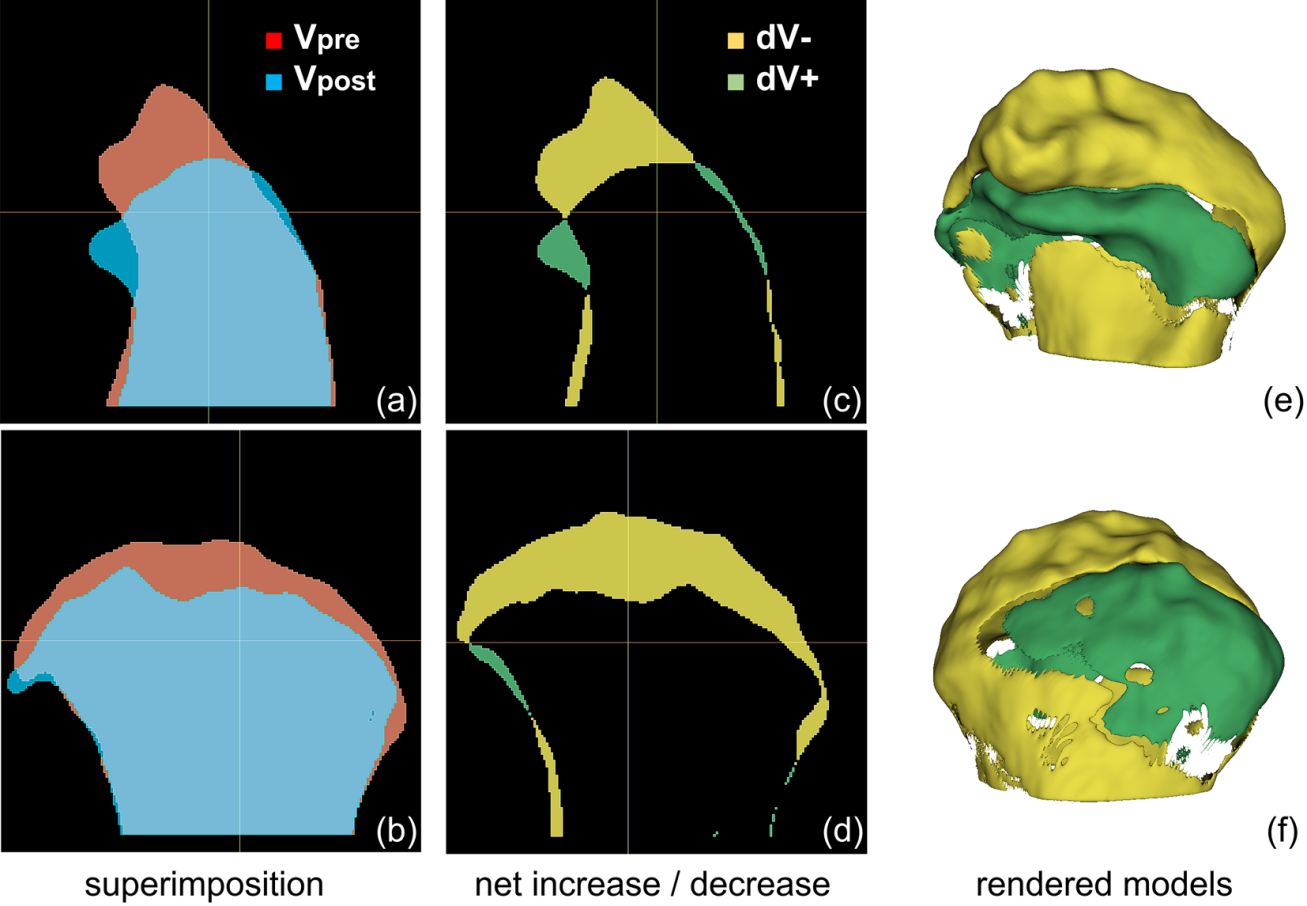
Representative volumetric analysis of processed cone-beam computed tomography (CBCT) images. (a–b) Superimposition of Vpre and Vpost in sagittal and coronal view. (c–d) Sagittal and coronal views of net decrease (dV− ) and net decrease (dV +). Yellow segment, dV−  = Vpre− overlap; Green segment, dV +  = Vpost-overlap. (e–f) Anterior and posterior views of the 3D model of dV−  and dV + . The images were generated using the open-source software 3D Slicer (v4.11, Slicer, http://www.slicer.org/).
